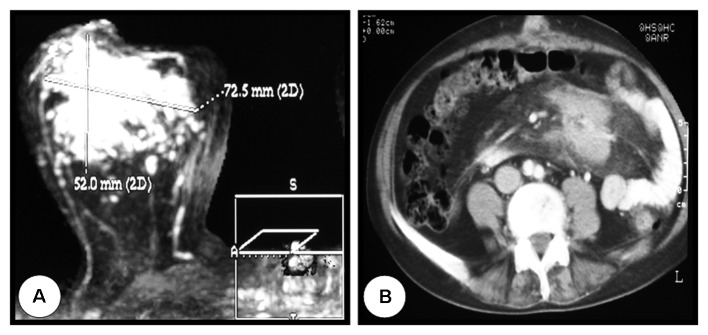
Synchronous solid neuroendocrine breast carcinoma and abdominal lymphoma. (A) Magnetic resonance image (MRI) showing a 7-cm mass that is highly suggestive of neuroendocrine breast carcinoma (NEBC) in the upper outer quadrant of the patient’s right breast; (B) Computed tomography (CT) scan of the abdomen showing a mesenteric mass of 6 cm (i.e., synchronous abdominal non-Hodgkin’s lymphoma).
